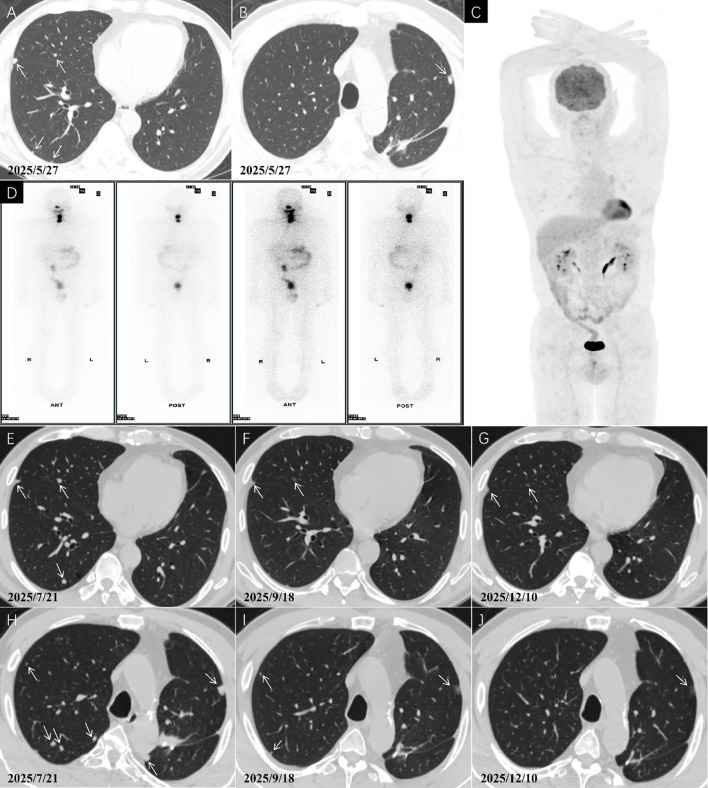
Results of the patient’s ^18^F-FDG PET/CT, post-therapeutic (200mCi ^131^I) whole-body scan (WBS), and follow-up chest CT scans. The patient’s initial post-operative PET/CT scan revealed progression of multiple PMs (white arrow in (A, B)), with the largest measuring approximately 8.0mm in diameter (white arrow in (B)), and no evidence of additional metastatic disease (C). Subsequently, a therapeutic dose of ^131^I showed no uptake in these PMs (D). Follow-up chest CT scans conducted before and after the initiation of targeted combined with immunotherapy demonstrated further progression (white arrow in (E, H)) compared to the pre-radioiodine therapy scan (white arrow in (A, B)), with the largest lesion increasing to approximately 10.4mm (white arrow in (H)). However, subsequent evaluations following systemic therapy indicated a positive treatment response, with the PMs decreasing in both size and number (white arrow in (F, G, I, J)). The largest lesions measured approximately 8.1mm and 7.8mm on successive scans (white arrow in (I, J)).
